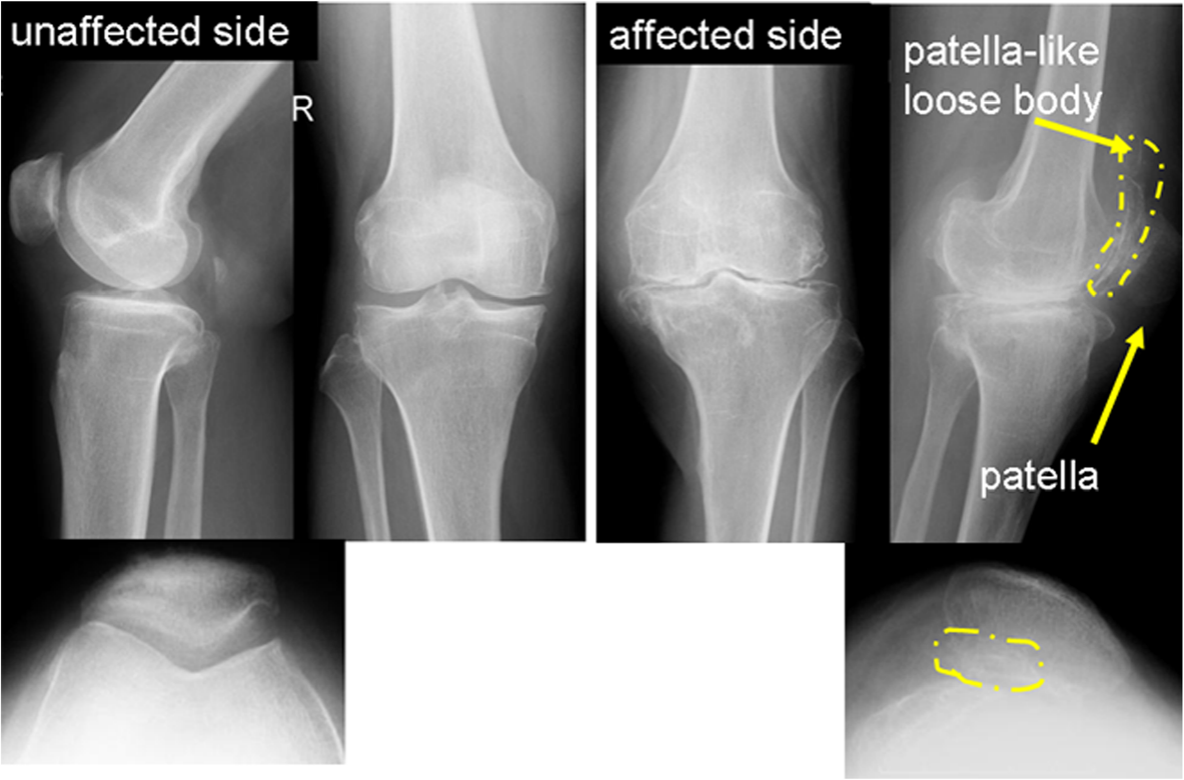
X-ray analysis. X-ray analysis showed a bone fragment in the patellofemoral joint.
